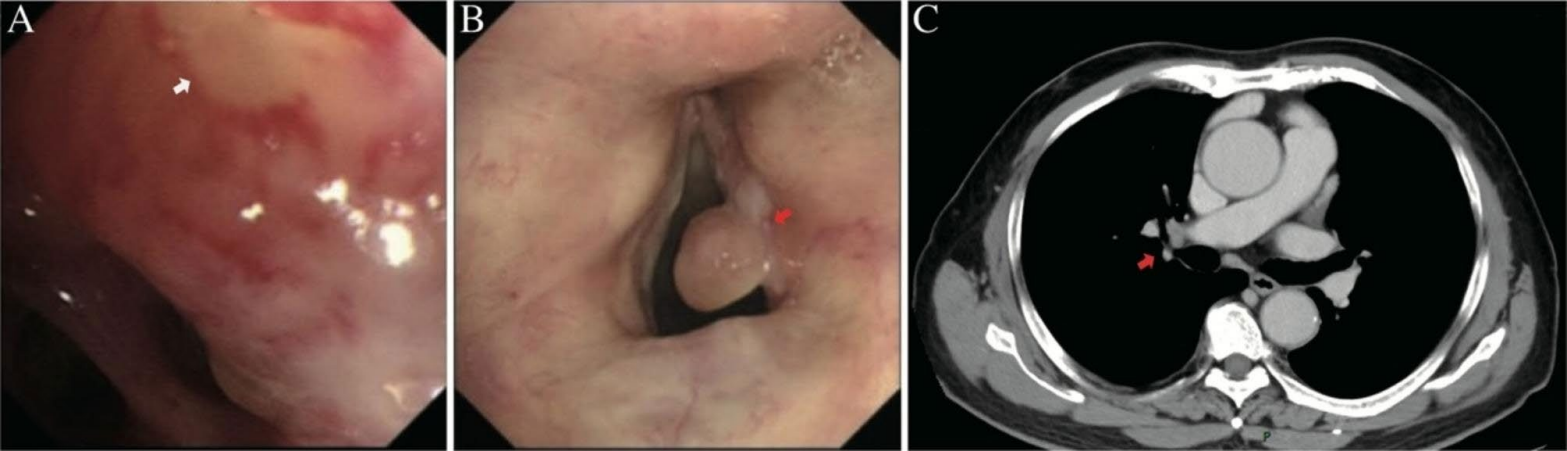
Preoperative representative radiologic and bronchoscopic images from the current case. (A) Bronchoscopic findings show a neoplasm arising from the opening of the posterior segmental bronchi of the right upper lobe (arrow). (B) Laryngoscope findings showing a polyp on the posterior portion of the right vocal fold with leukoplakia (arrow) (C) Computed tomography scan showing a well-demarcated irregular mass in the posterior segmental bronchial root of the posterior apex of the upper right lung (arrow)
